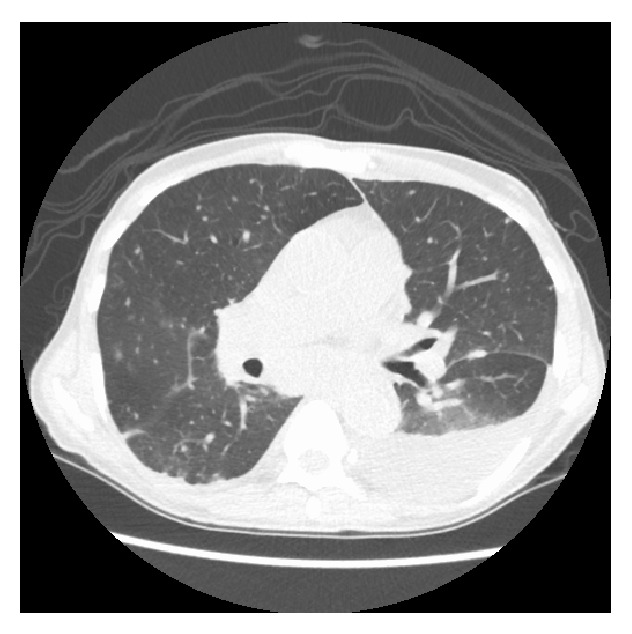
CT scan of chest showing diffuse lung nodules bilaterally, left sided effusion, and mediastinal adenopathy.
